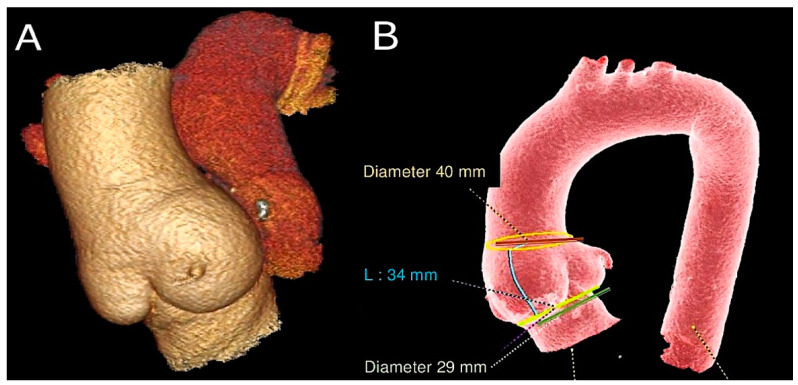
This CT scan shows (A) pulmonary autograft and pulmonary homograft from a Ross operation performed 23 years ago. (B) Measurements of PA were taken at the sinotubular junction, sinuses of Valsalva, and aortic annulus [51,52].
